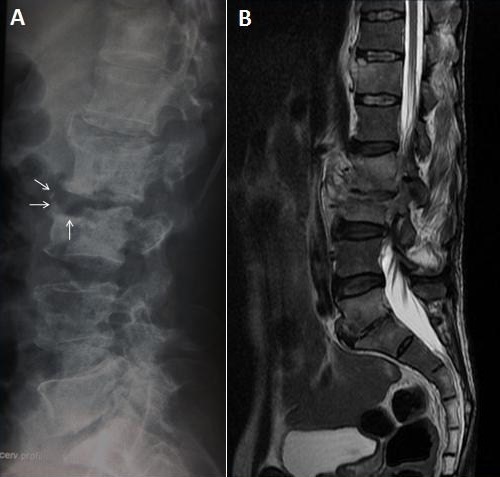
Radiography of the spine revealed parrot's peak appearance (Pedro Pons’ sign) at the anterior superior end of the L3 vertebra which is characterized by osteosclerosis and osteophyte (A, arrows). Magnetic resonance imaging (MRI) after the administration of gadolinium revealed L2-L3 epiduritis, an erosive change of the anterior portion of L4 and L5, abnormal signal change with enhancement in T12, L2, L3, L5 and S1 body with probable spondylodiscitis in L2/L3 and L5/S1 levels (B).
